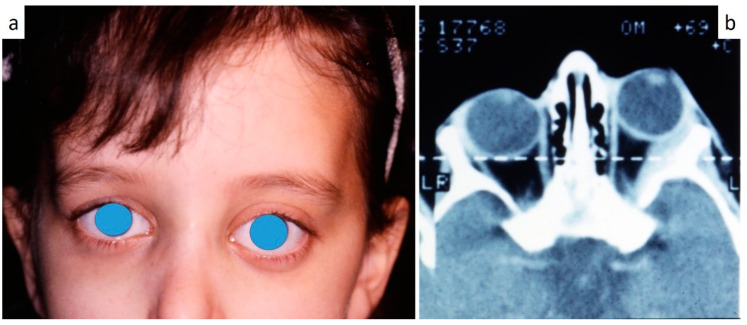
(a,b): Exophthalmia (a) in LCH patient as confirmed by CT scan (b).
